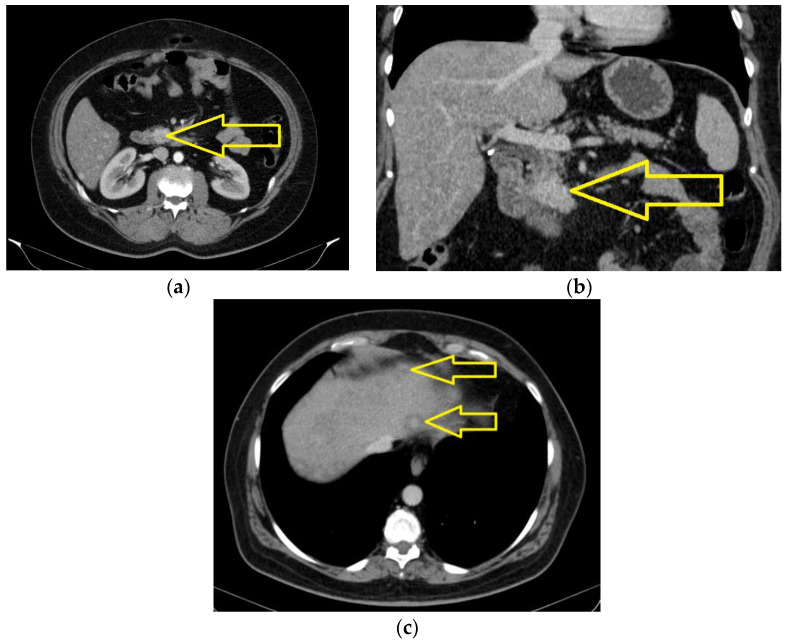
The computed tomography of the abdomen with intravenous contrast medium (May 2023). An expansive polylobate process of approximately 21.4/27 compared to 25/27 mm axially at the previous examination: axial section (a) and coronal section (b) (yellow arrows); (c) liver of increased dimensions (the cranial–caudal diameter of the LDH is 17.3 cm), spontaneously hypodense focal lesions disseminated in both hepatic lobes; some of them with a central contract uptake, the largest of them located in the IV B segment, with a maximum diameter of approximately 20/14 mm—known secondary hepatic determination in numerical progression (yellow arrows).
